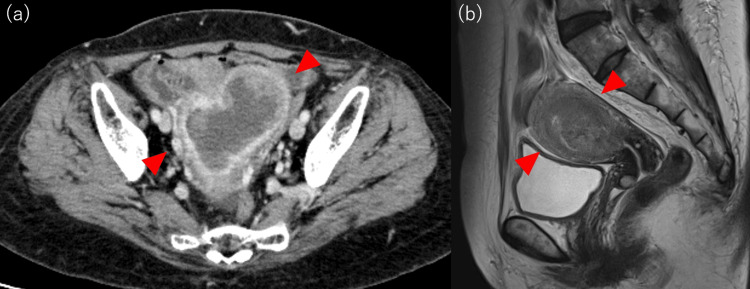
Preoperative imaging (a) A contrast-enhanced CT scan of the pelvis reveals a mass localized to the uterus, with no evidence of metastatic involvement (red arrowhead). (b) A T2-weighted MRI of the pelvis demonstrates a tumor confined to the uterine corpus, with an estimated invasion extending beyond 50% of the myometrium (red arrowhead) CT: computed tomography; MRI: magnetic resonance imaging
